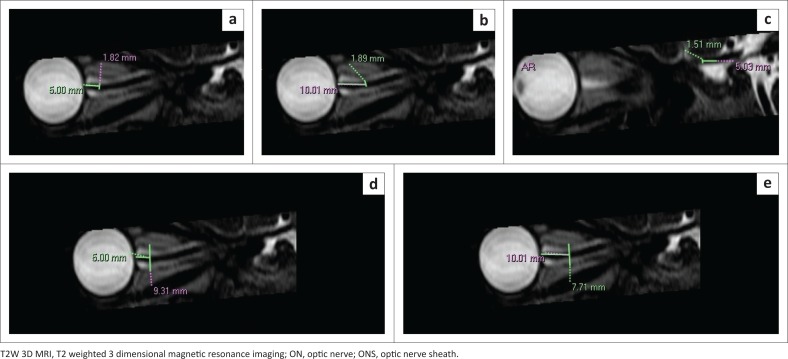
Reformatted sagittal T2W 3D magnetic resonance imaging of the right orbit demonstrating the measurement technique: (a and b) intraorbital optic nerve height 5 mm and 10 mm behind the globe, respectively; (c) intracranial optic nerve height and (d and e) optic nerve sheath height 5 mm and 10 mm behind the globe, respectively.
